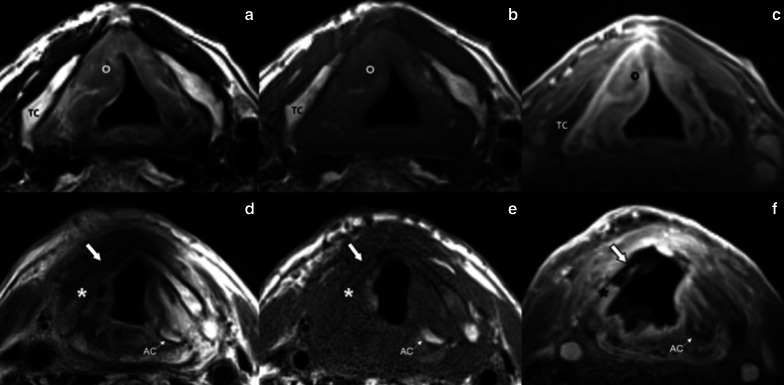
Laryngeal necrosis in an 88-year-old man five months after RT for a right glottic-supraglottic cancer. Surface-coil MRI of the larynx: pre-treatment axial T2-weighted (a), T1-weighted (b) and post-contrast 3D GRE fat-sat T1-weighted (c) MR images show a lesion of the right vocal fold (circle). Post-treatment axial T2-weighted (d), T1-weighted (e) and post-contrast 3D GRE fat-sat T1-weighted (f) show extensive necrosis of the right true vocal fold, anterior commissure and right lamina of the thyroid cartilage (asterisk); the right arytenoid cartilage is not recognizable. Cartilage fragmentation is pointed by the bold white arrow. TC, thyroid cartilage, AC, arytenoid cartilage
